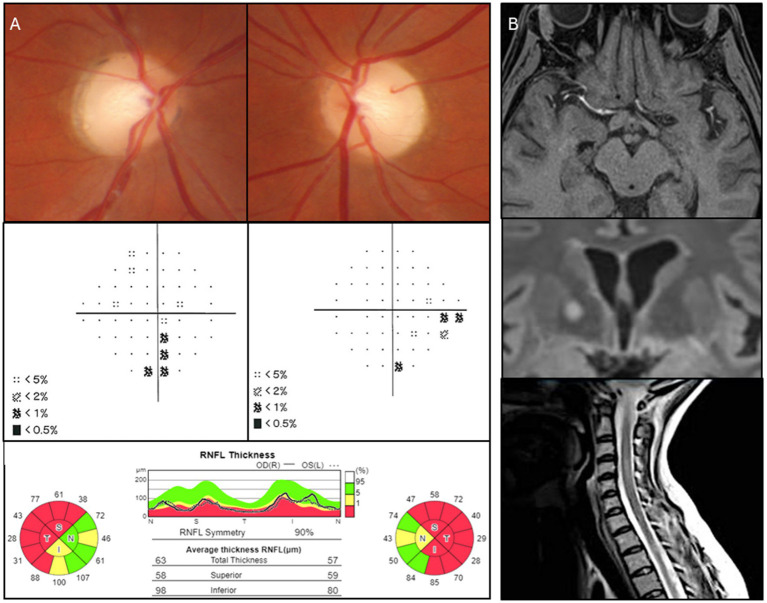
(A) Ophthalmological findings: in the upper panel fundoscopy shows bilateral optic disc pallor; in the middle panel visual fields (VF) show bilateral inferior defects; in the lower panel optical coherence tomography (OCT) shows bilateral thinning of the RNFL, with preservation of the nasal quadrant. (B) Radiological findings: in the upper panel axial T1-weighted Fat-Saturated brain MRI shows an optic glioma involving the right optic chiasma and right optic tract; in the middle panel coronal FLAIR T2-weighted brain MRI shows focal area of signal intensity (FASI) in the right globus pallidus; in the lower panel sagittal T2-weighted cervical spinal cord MRI shows multiple hyperintense demyelinating lesions.
